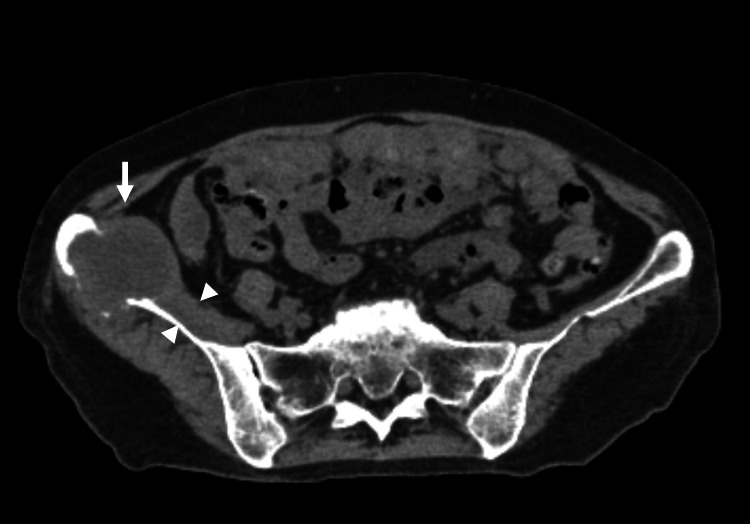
CT imaging at initial radiation oncology consultation CT: computed tomography The CT scan from the initial radiation oncology consultation reveals an enlarging mass in the right iliac bone with osteolytic bone metastasis (arrow). Additionally, the right psoas muscle appears hypertrophied compared to the left (arrowheads)
